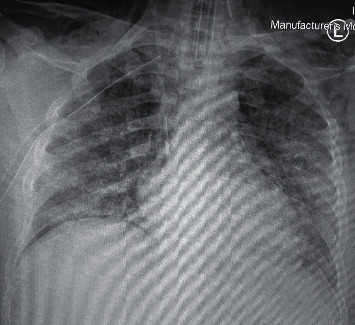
Admission chest X-ray showing bilateral extensive ground glass opacities.
